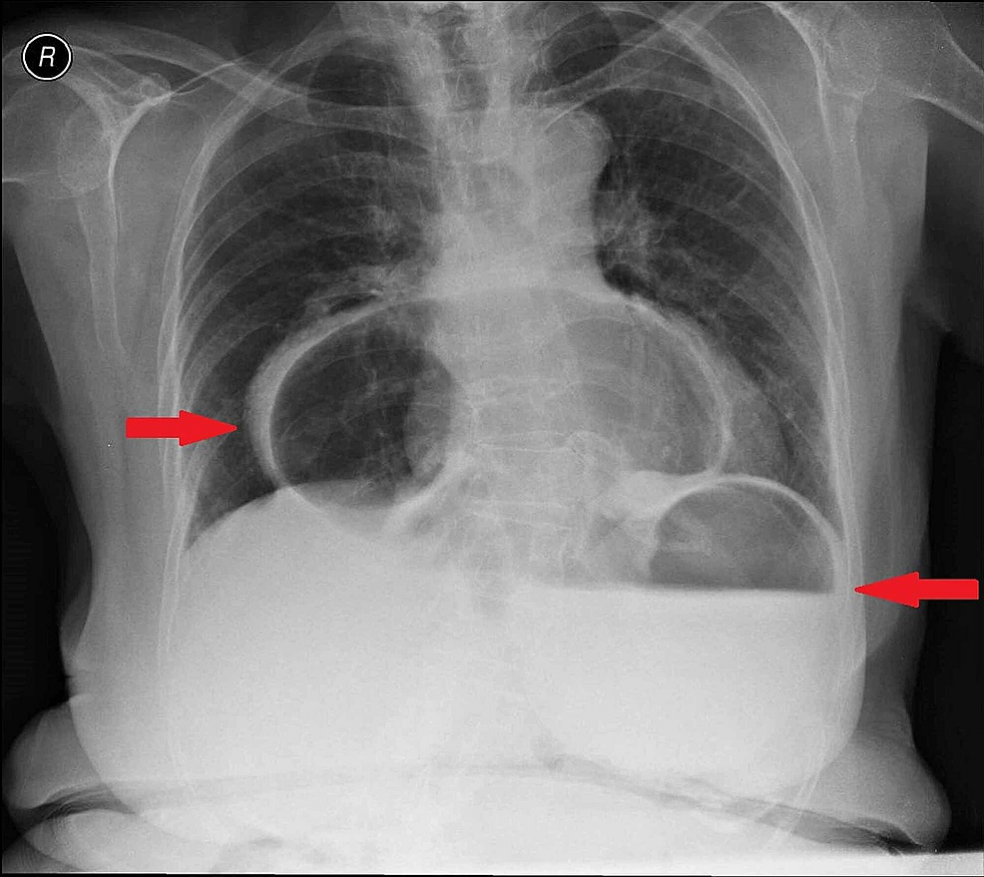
Chest radiography showing wide hernia of the hiatus June, 2016. The right arrow shows a stomach portion within the thoracic cavity and the left arrow shows another stomach portion within the abdominal cavity.
